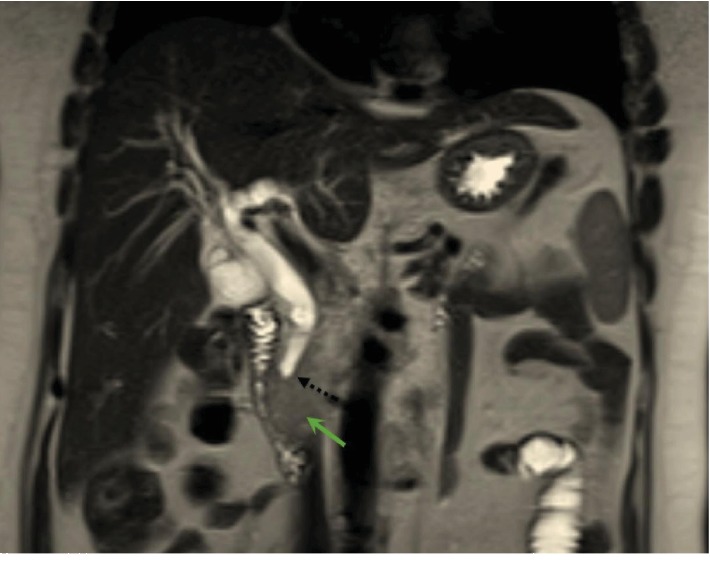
Magnetic resonance imaging (MRI) of the abdomen. Coronal slice obtained through the second part of the duodenum (D2) shows the uncinate process mass (green arrow) obstructing the distal common bile duct (dotted black arrow).
